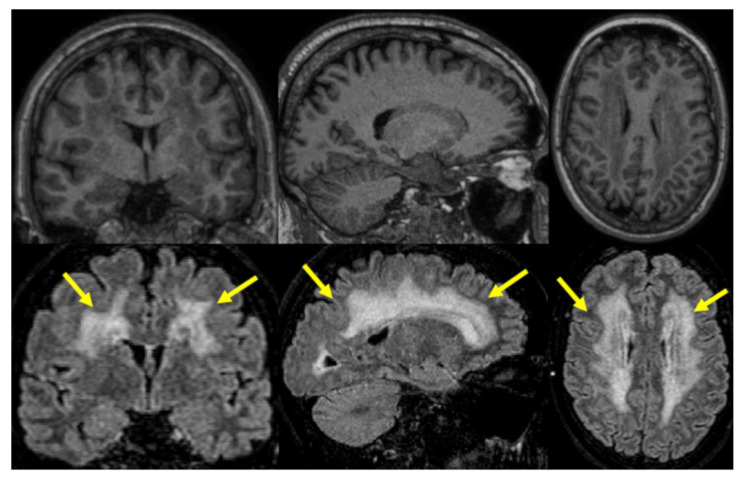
Representative cerebral MRI-T1 (top) and MRI-FLAIR (below) images from a 50-year-old Skogholt patient. Note the extensive white matter hyperintensities (WMHs) typical of the disease (see arrows).
